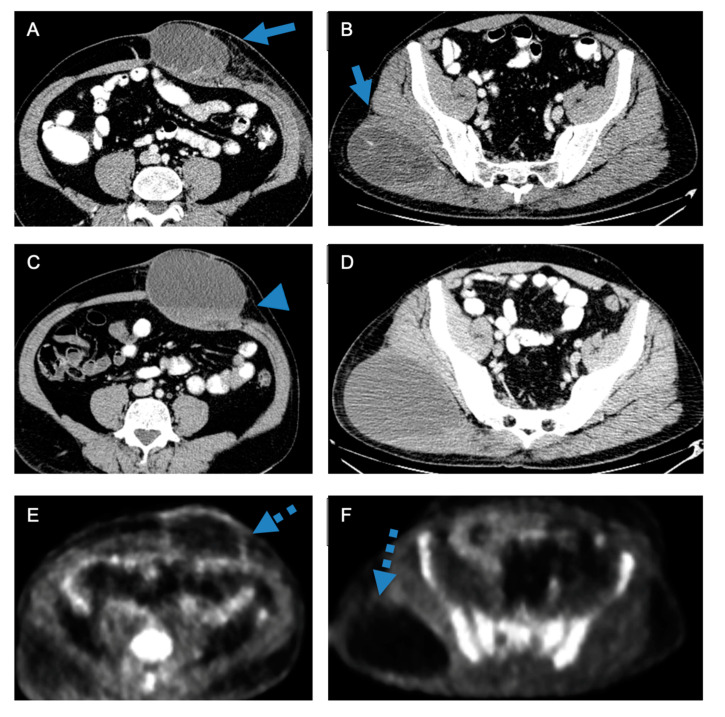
Discrepancy between radiology report and multidisciplinary tumor board assessment. A 55-year-old man with a metastatic high-grade undifferentiated pleomorphic sarcoma. Axial CT images (A,B) obtained at the time of diagnosis show two hypodense masses in the right posterior gluteal region and in the anterior abdominal wall (arrows). Axial CT images (C,D) obtained after 4 cycles of gemcitabine/taxotere show the mildly increased size of the masses but a decreased internal density and areas of fluid–fluid level within the anterior abdominal wall mass (arrowhead). The radiology report suggested the progression of the disease given the increase in the size of the lesions. The case was reviewed at a multidisciplinary tumor board and the consensus was that the increased tumor size was likely due to increased necrosis and possibly internal bleeding, suggesting a response to treatment. The findings were interpreted as stable disease. The patient was kept on gemcitabine/taxotere. Follow-up PET images (E,F) show only mild peripheral FDG uptake within the two masses (dashed arrows), suggesting a response to treatment.
